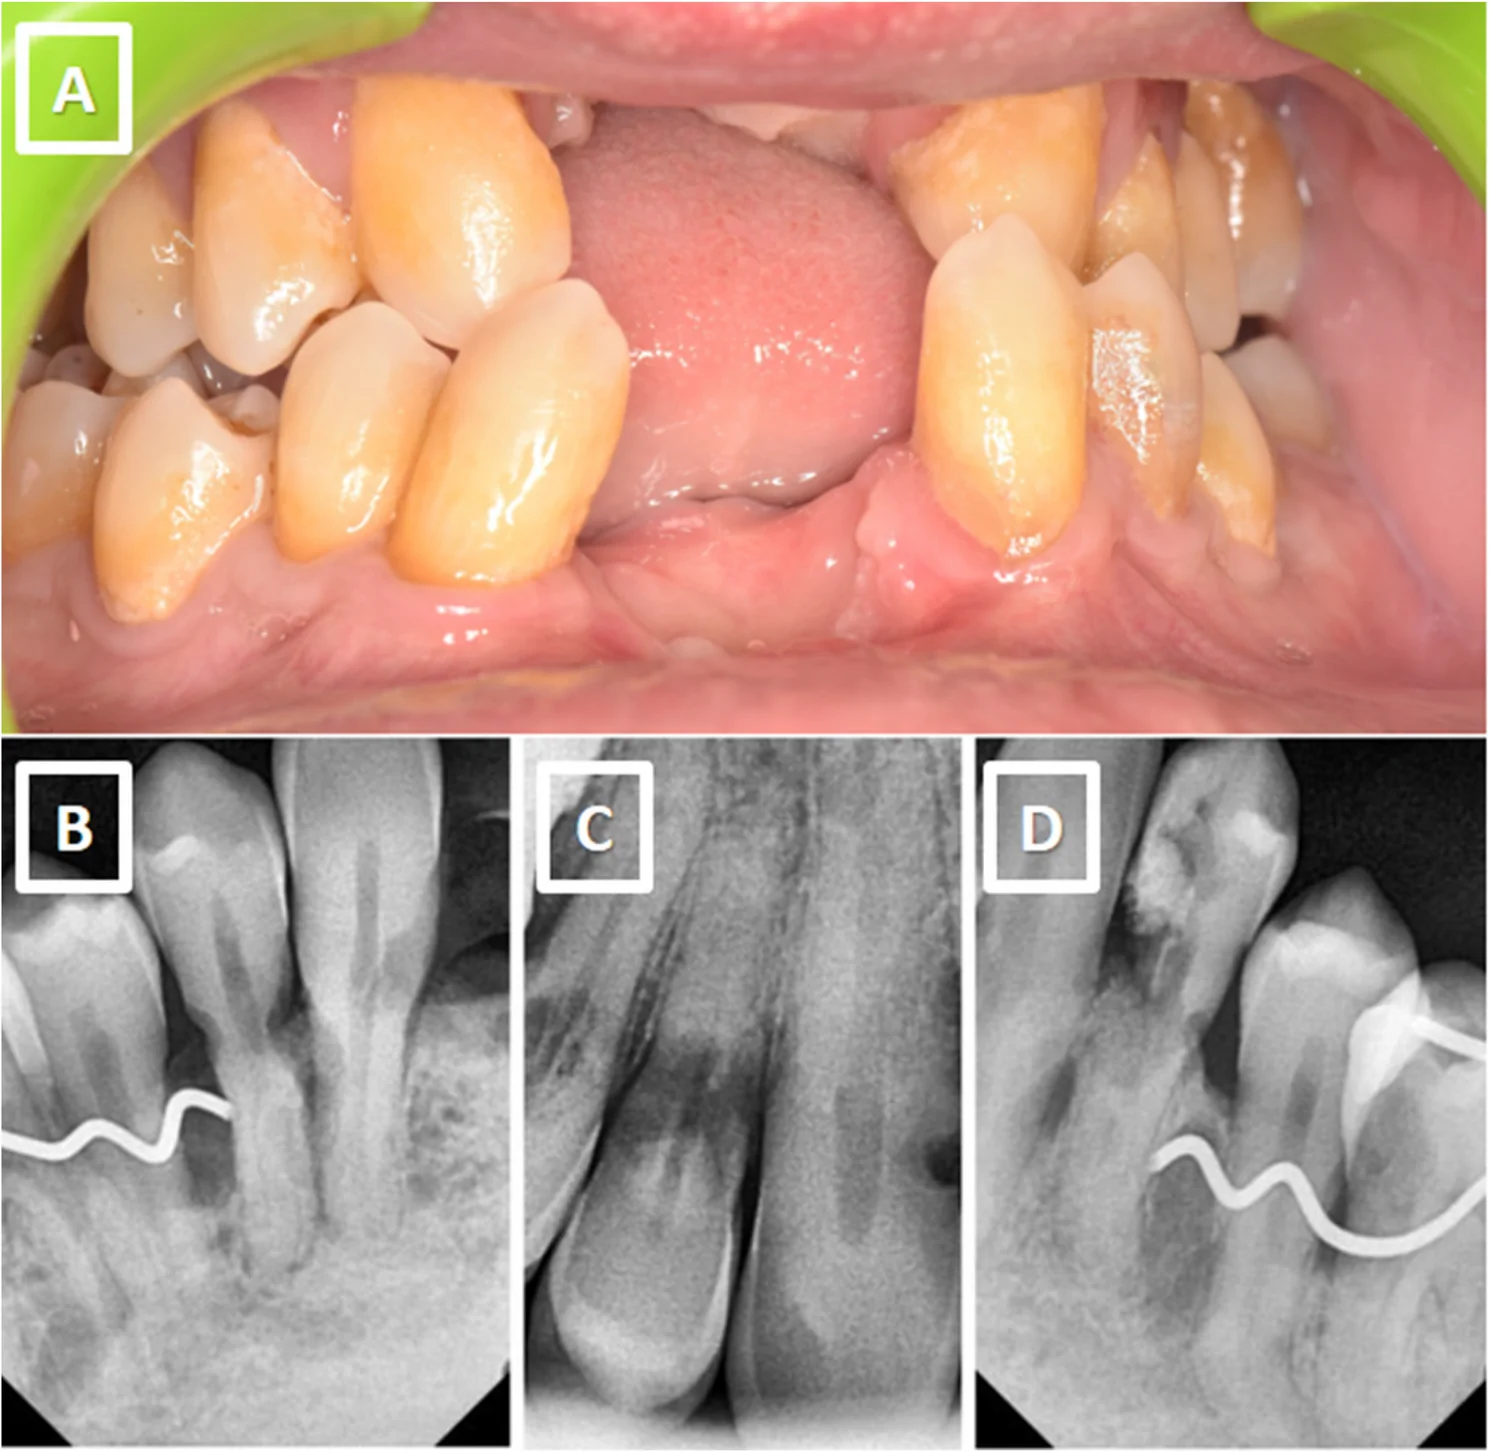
A Intraoral photograph at initial examination showing missing teeth and malocclusion. B–D Periapical radiographs demonstrating external root resorption with variable morphologies
